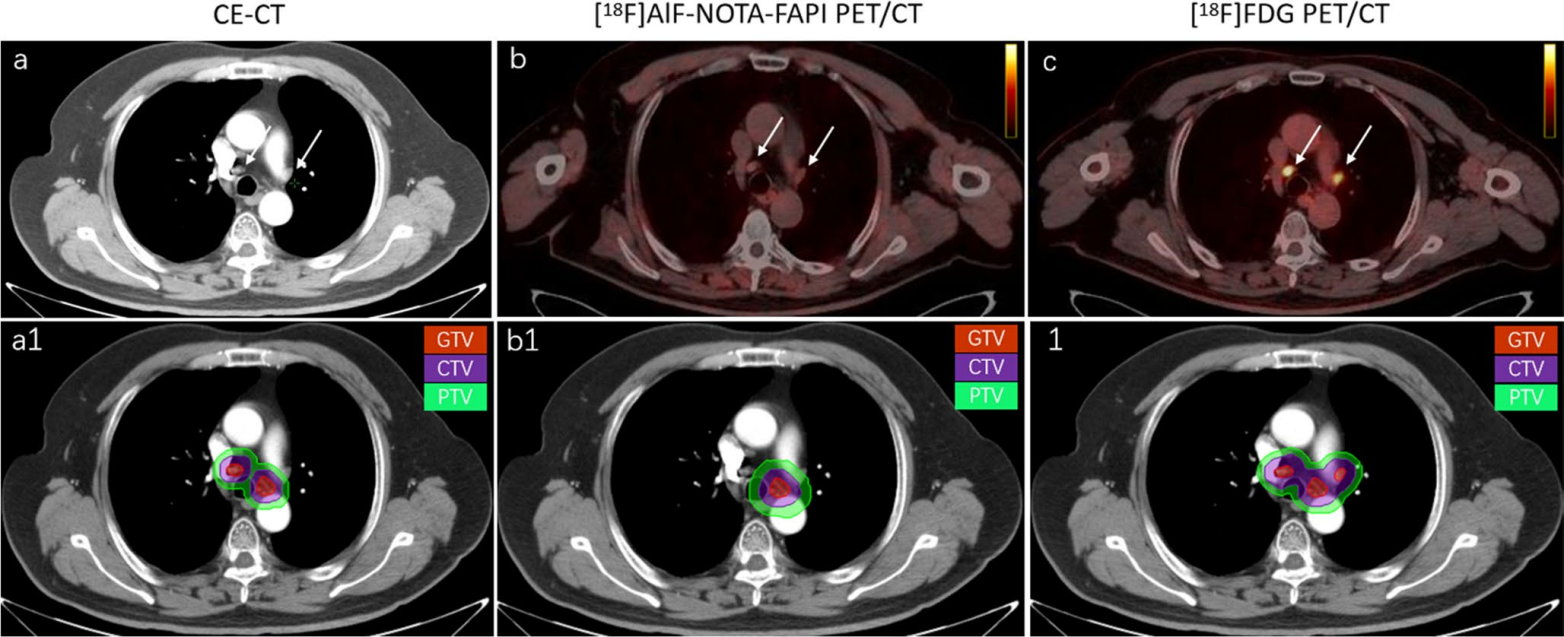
CE-CT, [^18^F]AlF-NOTA-FAPI PET/CT and [^18^F]FDG PET/CT scans of a 55-year-old man. 4R and 5 station mediastinal lymph nodes were diagnosed as true negative by CE-CT and [^18^F]FDG PET/CT, but false positive by [^18^F]FDG. Small-volume GTVnd, CTVnd and PTVnd can be delineated with the guidance of CE-CT and [^18^F]AlF-NOTA-FAPI, while larger target volume than the true lymph node was be delineated with the guidance of [^18^F]FDG PET/CT. White arrow points to lymph nodes
